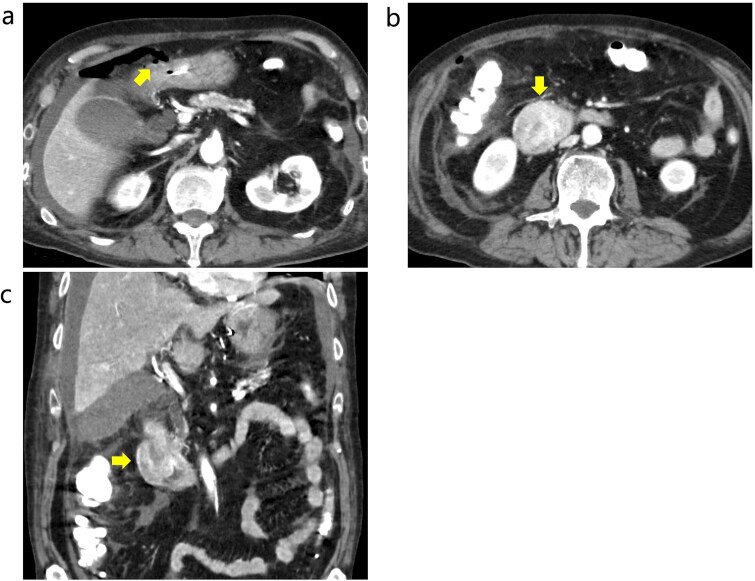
Contrast-enhanced CT before emergency surgery. (a) Free air was observed around the anterior wall in the duodenal bulb, and a perforation in the 1st portion was notes. The arrow indicates free air. (b and c) The tumor in the 2nd portion of duodenum was well enhanced. The arrows indicate the tumor.
